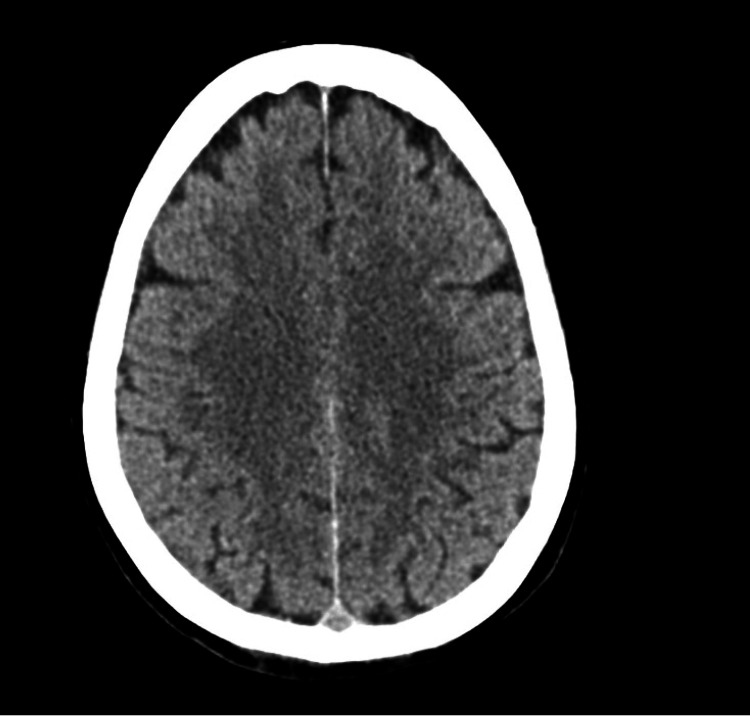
Initial computed tomography of the head showed no signs of any lesion
